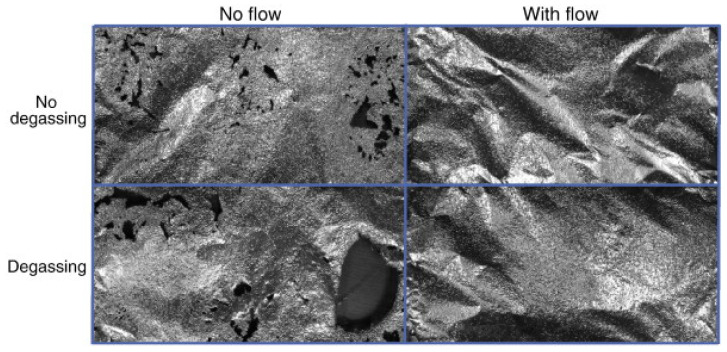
The damage to aluminium foil treated for 30 s in ultrasonic washing channel in various working conditions. Reprinted from [18] with permission.
